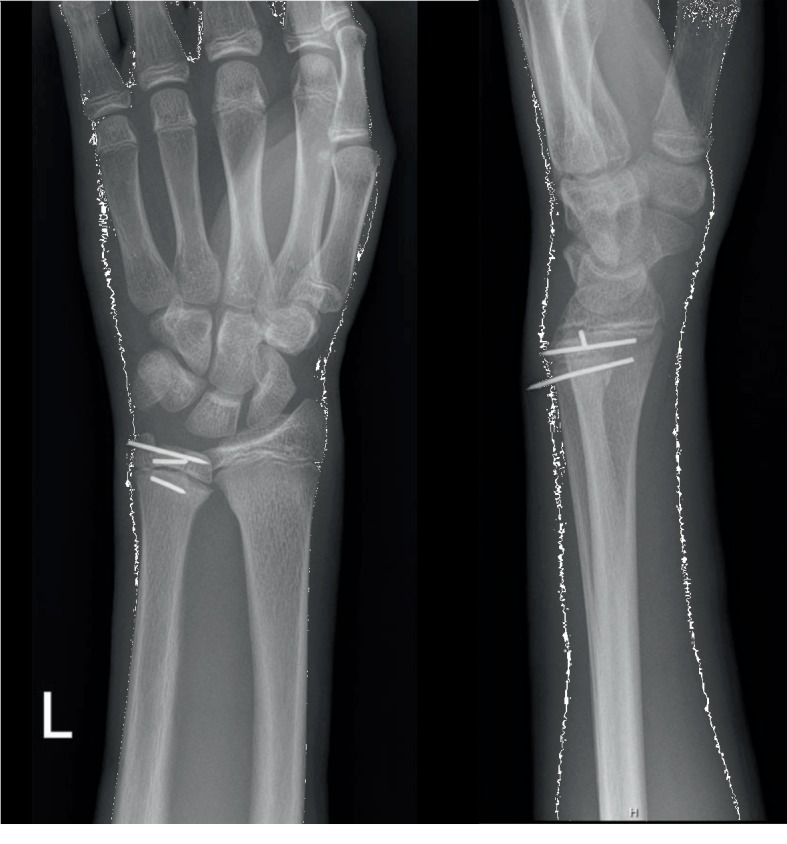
Anteroposterior and lateral X-ray 2 weeks after surgery.
